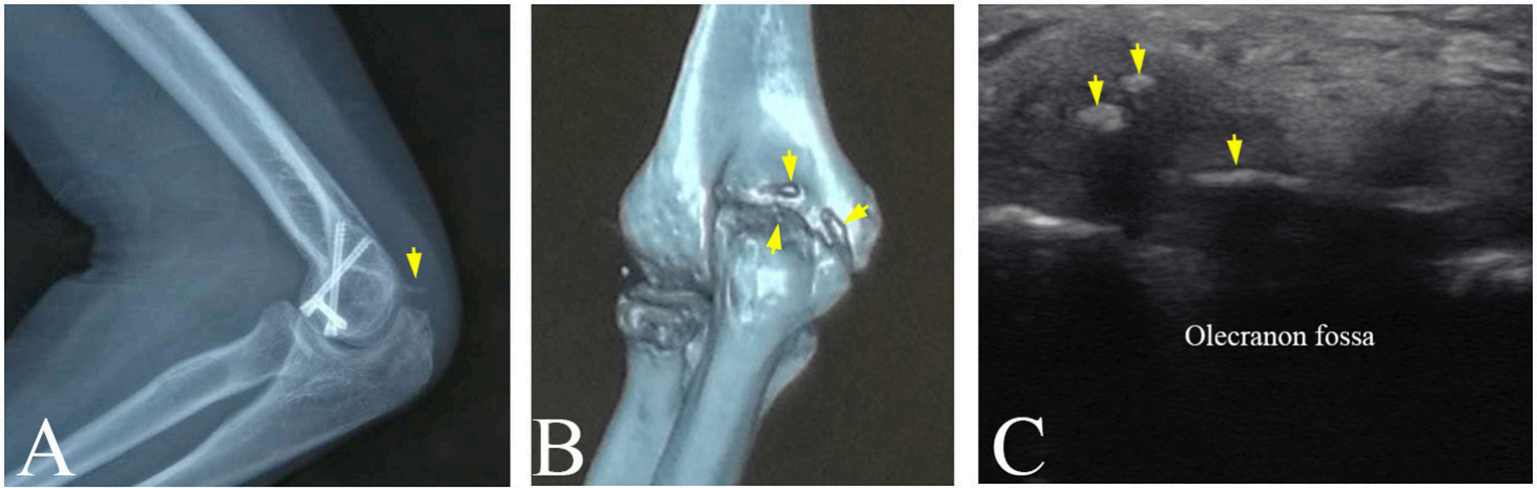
The HO tissues at left elbow joint of participant No. 4 visualized by multi-modality imaging. (A) A spiny HO (arrow) in plain radiograph. (B) A slender HO and two small and granular HOs discovered in CT image (arrows). (C) Two small hyperechoic masses and a slender hyperechoic region (arrows) in triceps brachii in ultrasound image.
